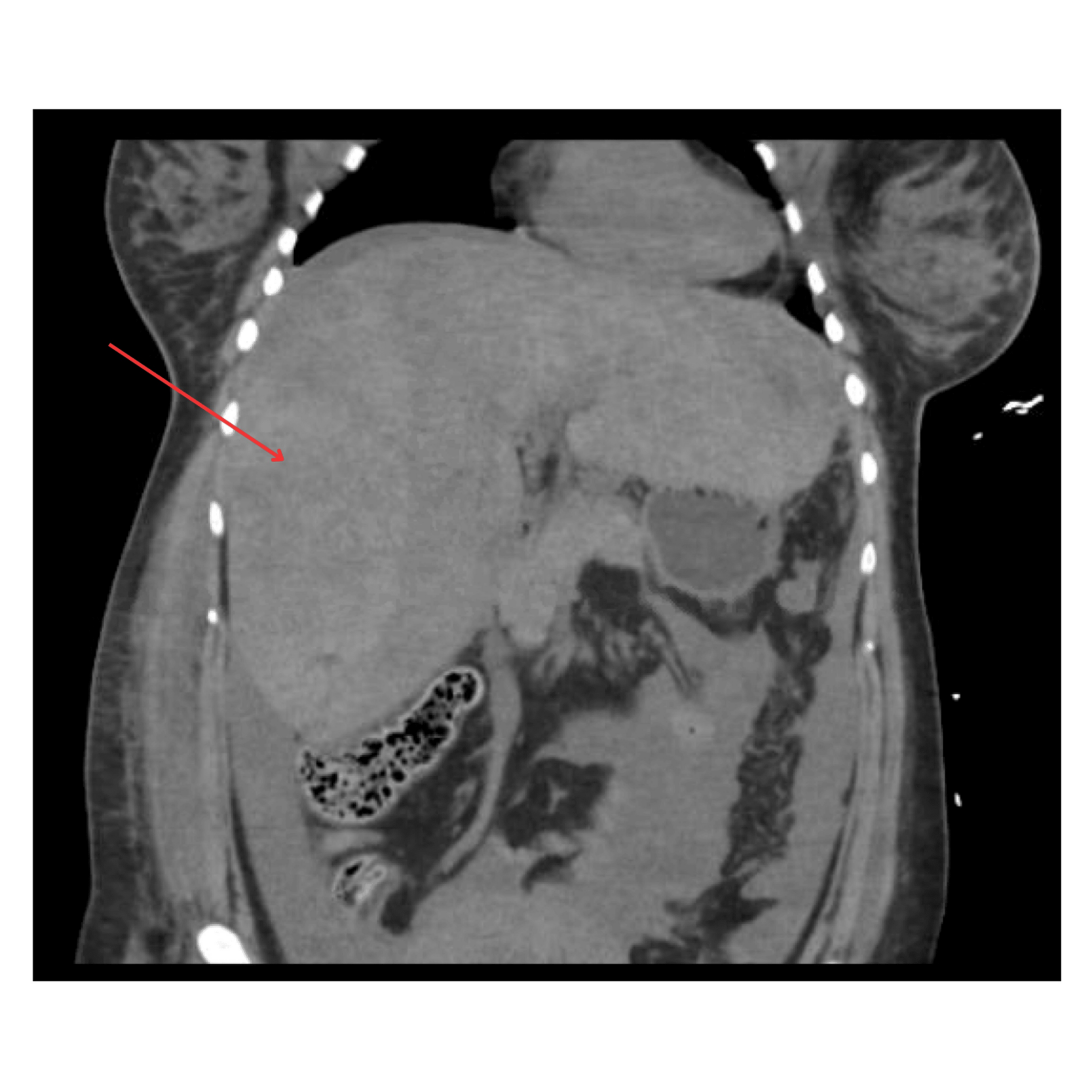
CT abdomen CT: computed tomography (CT) Large subcapsular hematoma seen along the anterior margin of the entire liver. This measures approximately 6.4cm in the greatest thickness along the right hepatic lobe.
